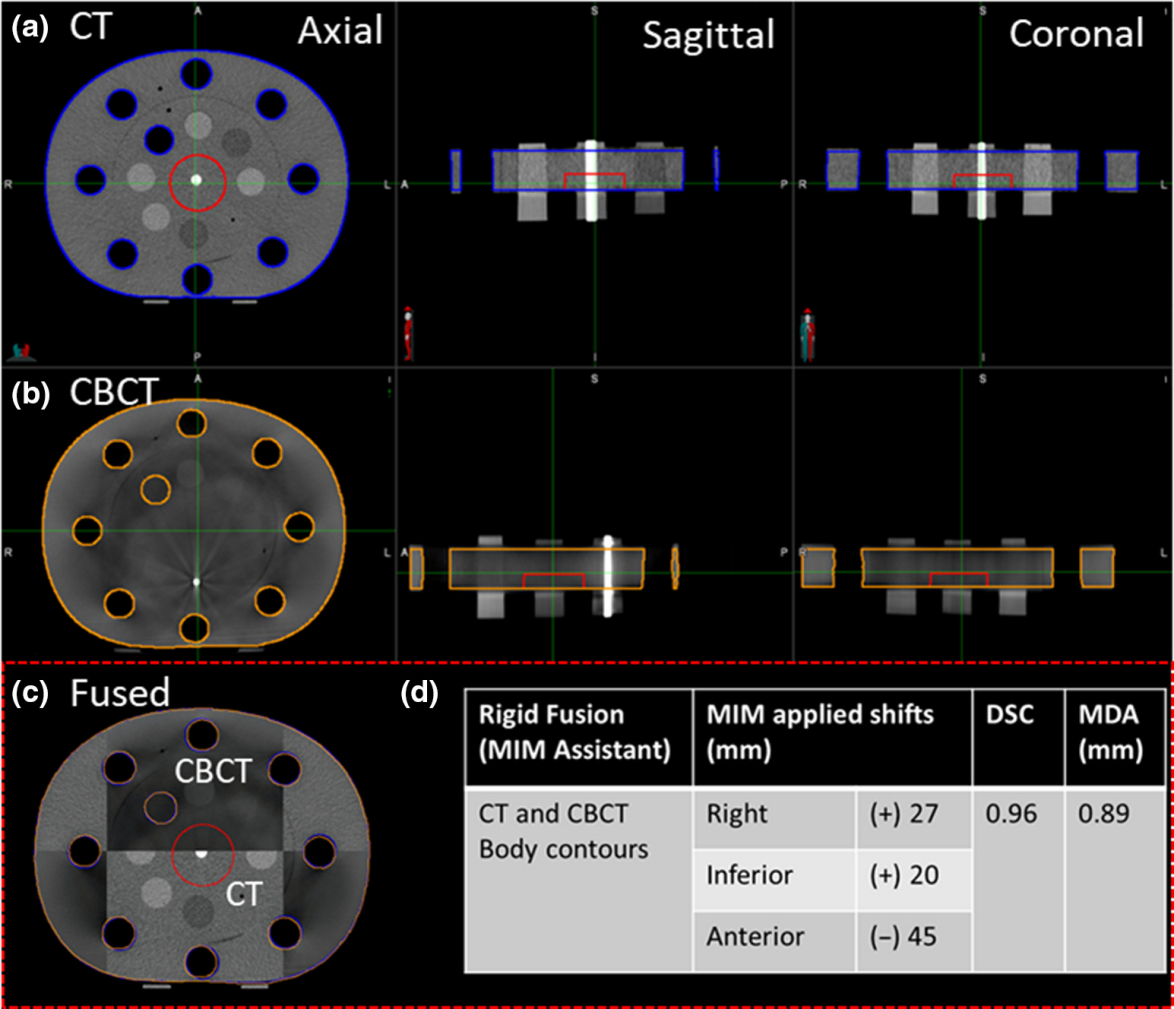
(A) CT body contour (Blue) and (B) CBCT body contour (orange) in three planes. (C) Checker‐box displaying the overlay between CT and CBCT images. (D) Rigid fusion results measured using DSC and MDA.
